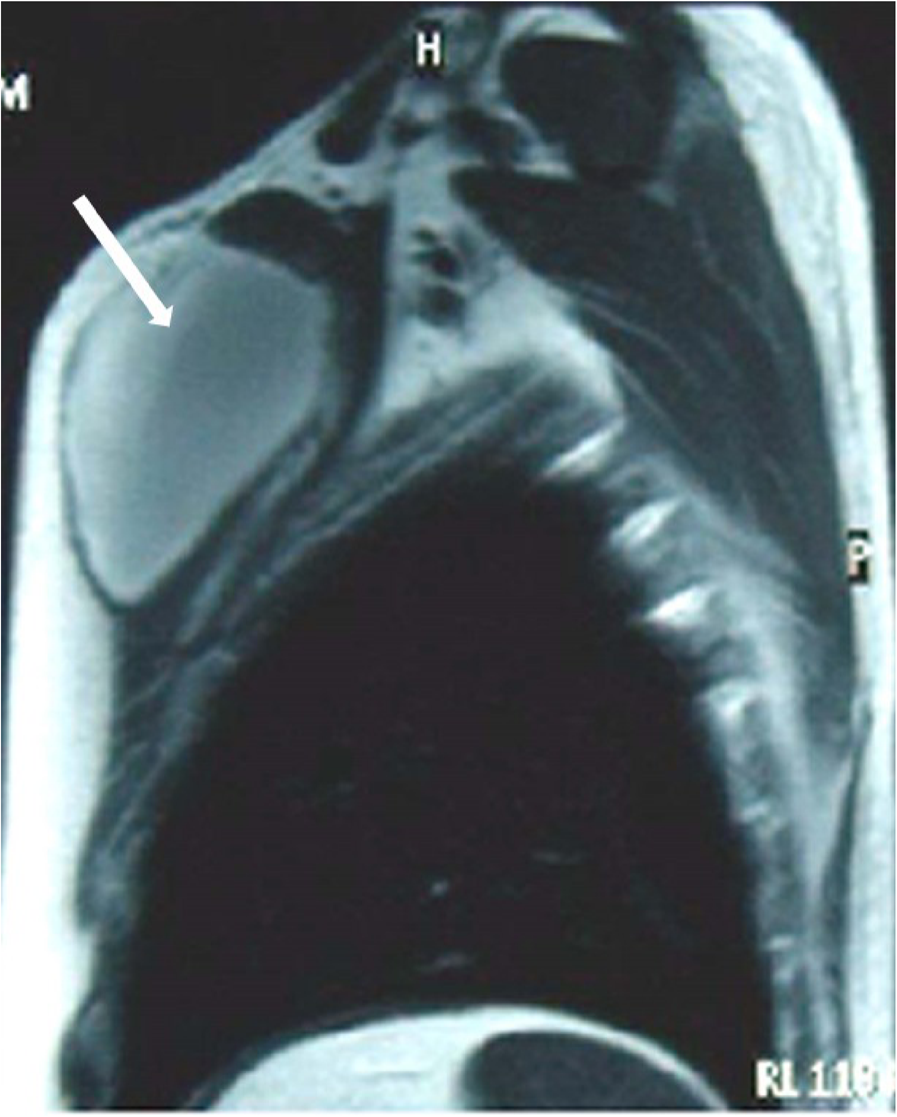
Magnetic resonance image: the arrow shows a large collection of pus in the chest wall located between the body and the manubrium of the sternum.
